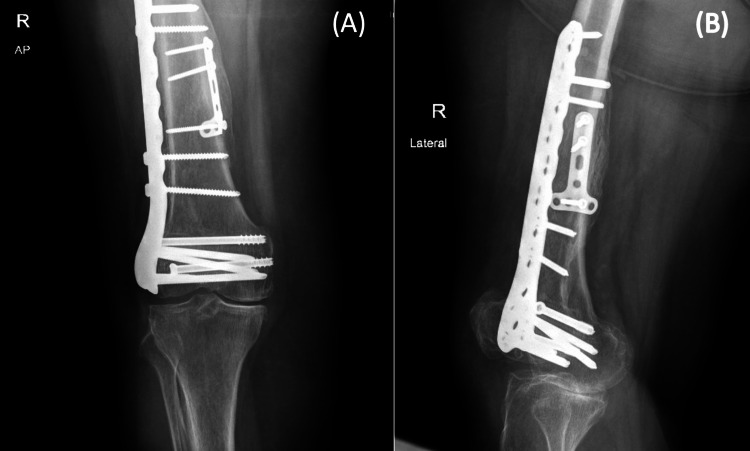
One-year follow-up X-rays (A) AP and (B) lateral views of the right knee joint. The one-year follow-up images show the healed fracture and proper alignment of the plate and screws.
